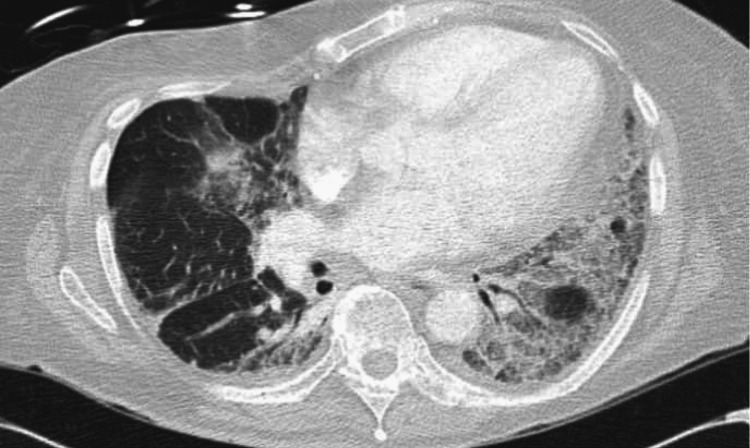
CT chest demonstrating interstitial lung disease which appears slightly worsened with possible developing honeycombing at the bases, superimposed ground glass opacities within the lung bases, right middle lobe, and lingula.
